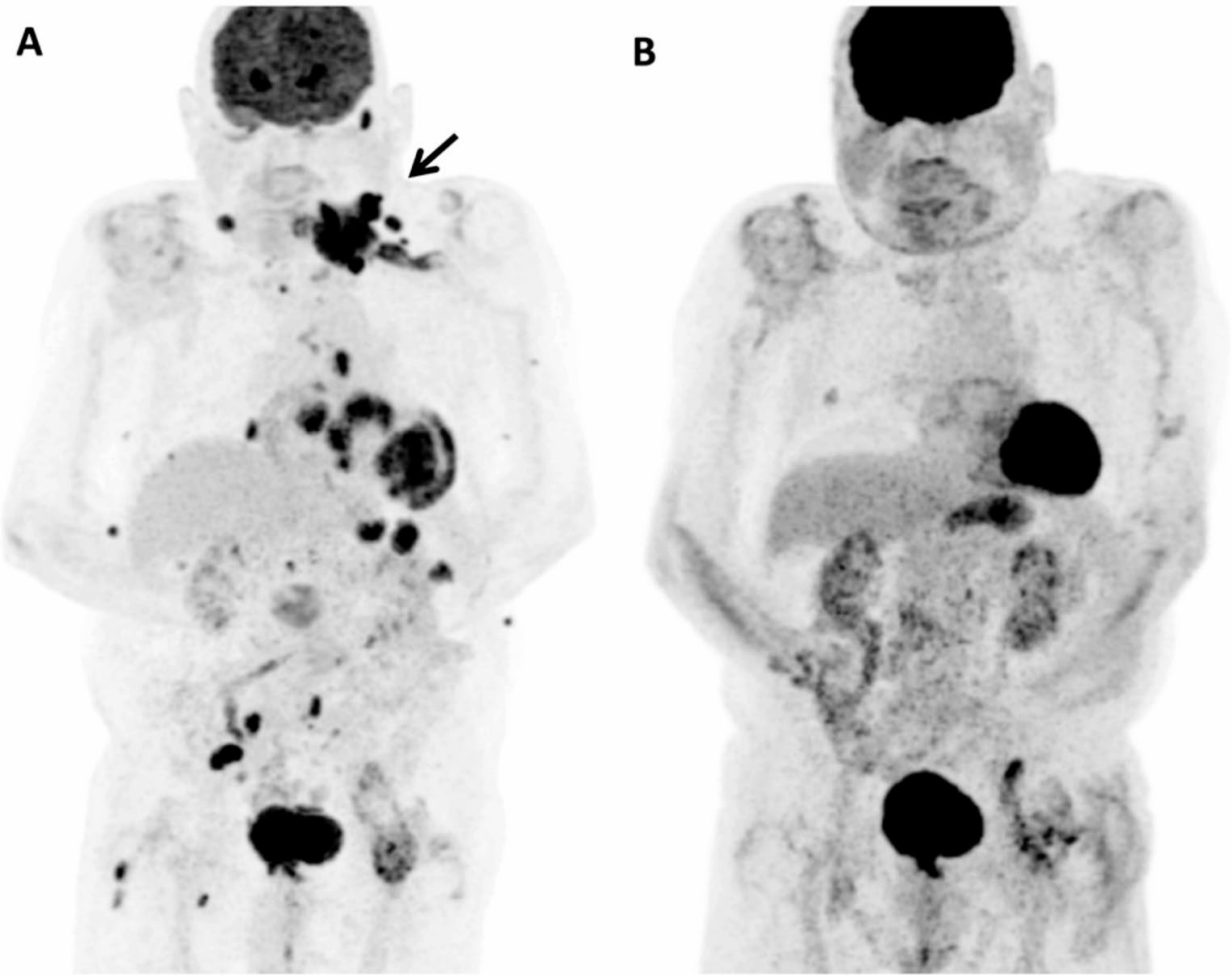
(A) 18 F-FDG PET MIP at relapse showing nodal and extra-nodal involvement at relapse. Bold arrow indicates lymph node cluster in the left supraclavicular region with intense hypermetabolism (SUV max 21.1) involving the 6th cervical vertebral body with extension to the left foraminal and spinal canal, left brachial plexus and nerves. (B) 18 F-FDG PET MIP after C9 showing a complete metabolic response
